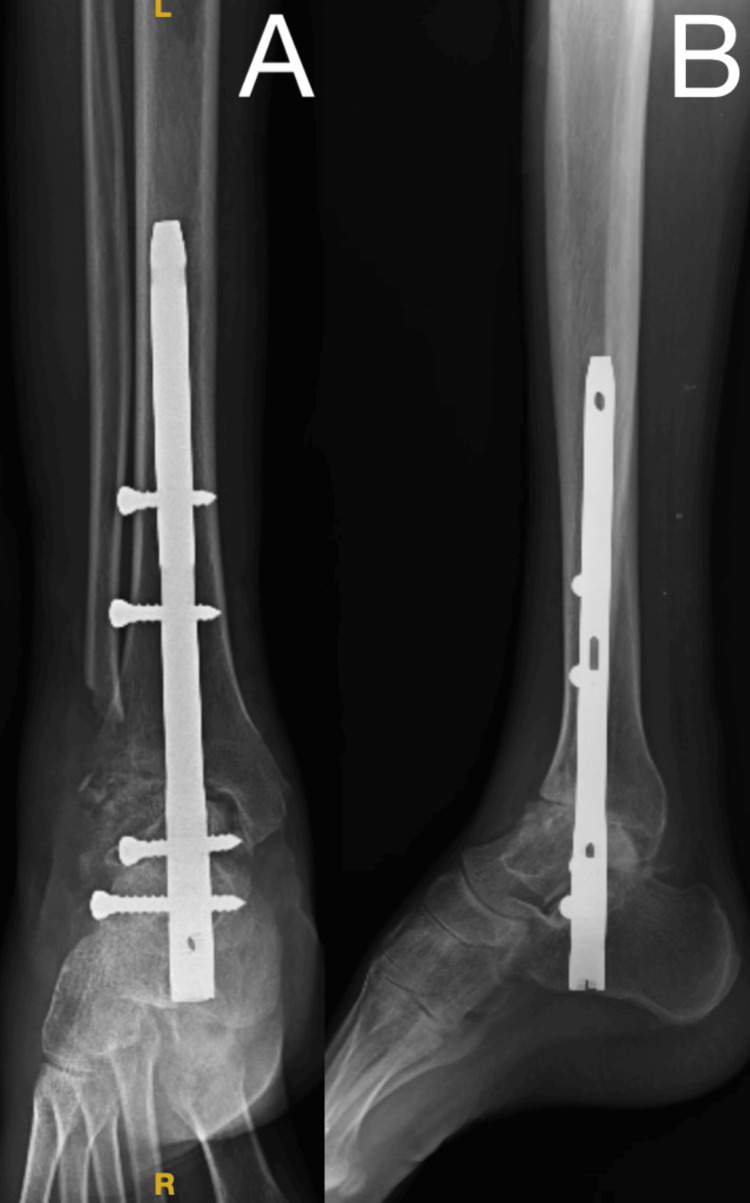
Postoperative radiograph of the right ankle (A) anteroposterior view and (B) lateral view
